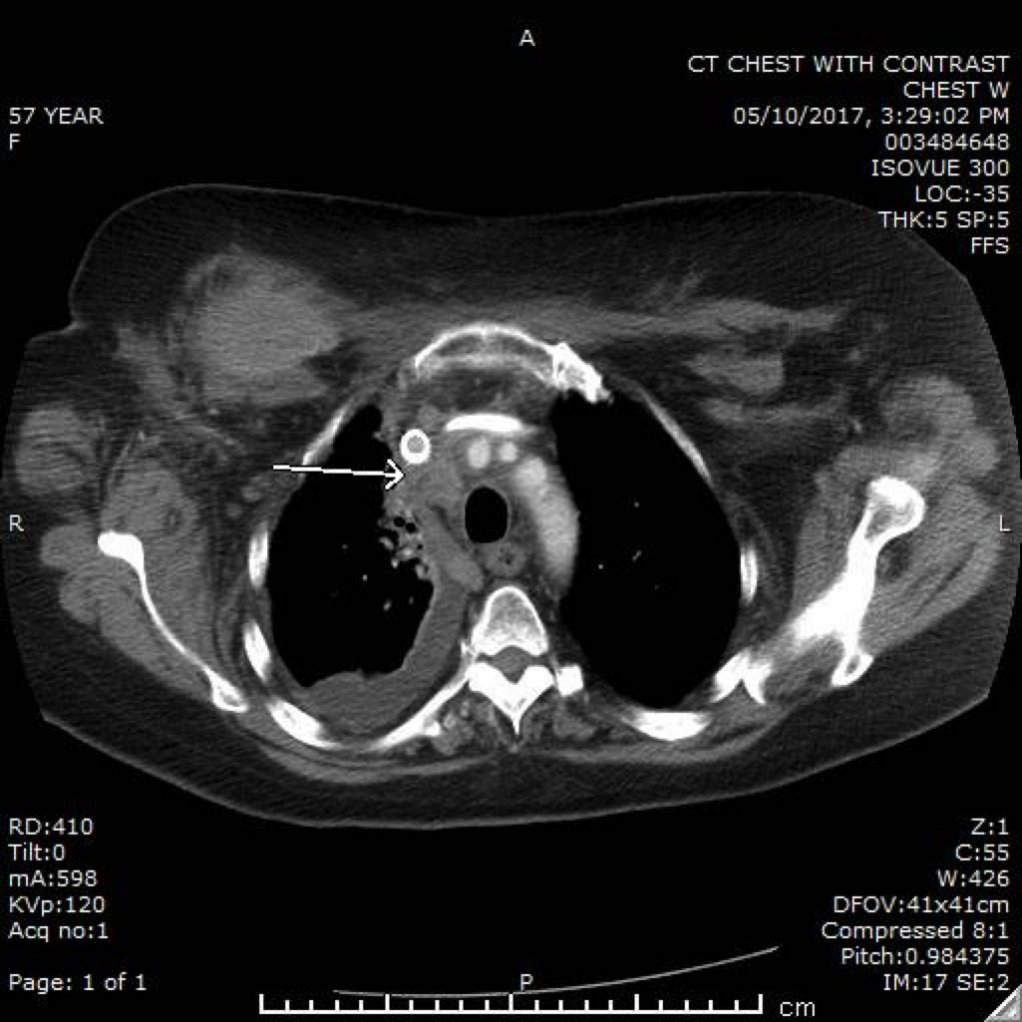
CT chest after six months of therapy on Olaparib showing improvement in the pleural effusion and lymphadenopathy
